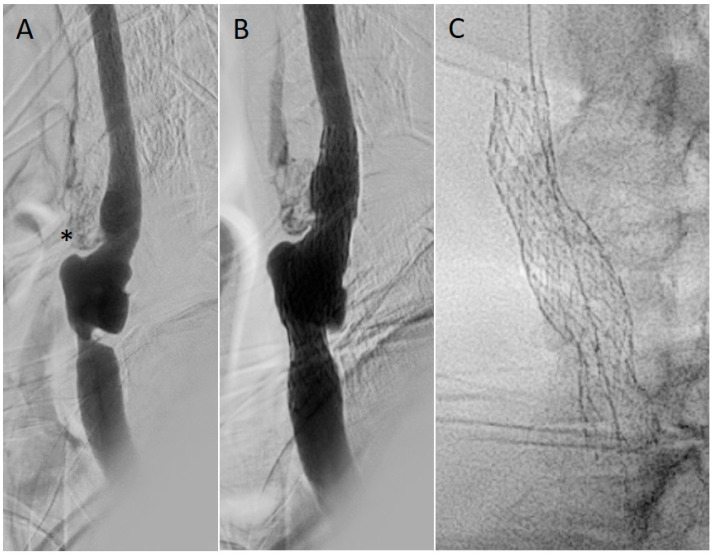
Treatment of an asymptomatic >80% restenotic lesion of the common carotid artery after previous carotid endarterectomy with Dacron patch plasty. (A) Angiography (* occlusion of external carotid artery); (B) completion angiography after dual-layer stent deployment; (C) radiographic aspect of dual-layer stent.
